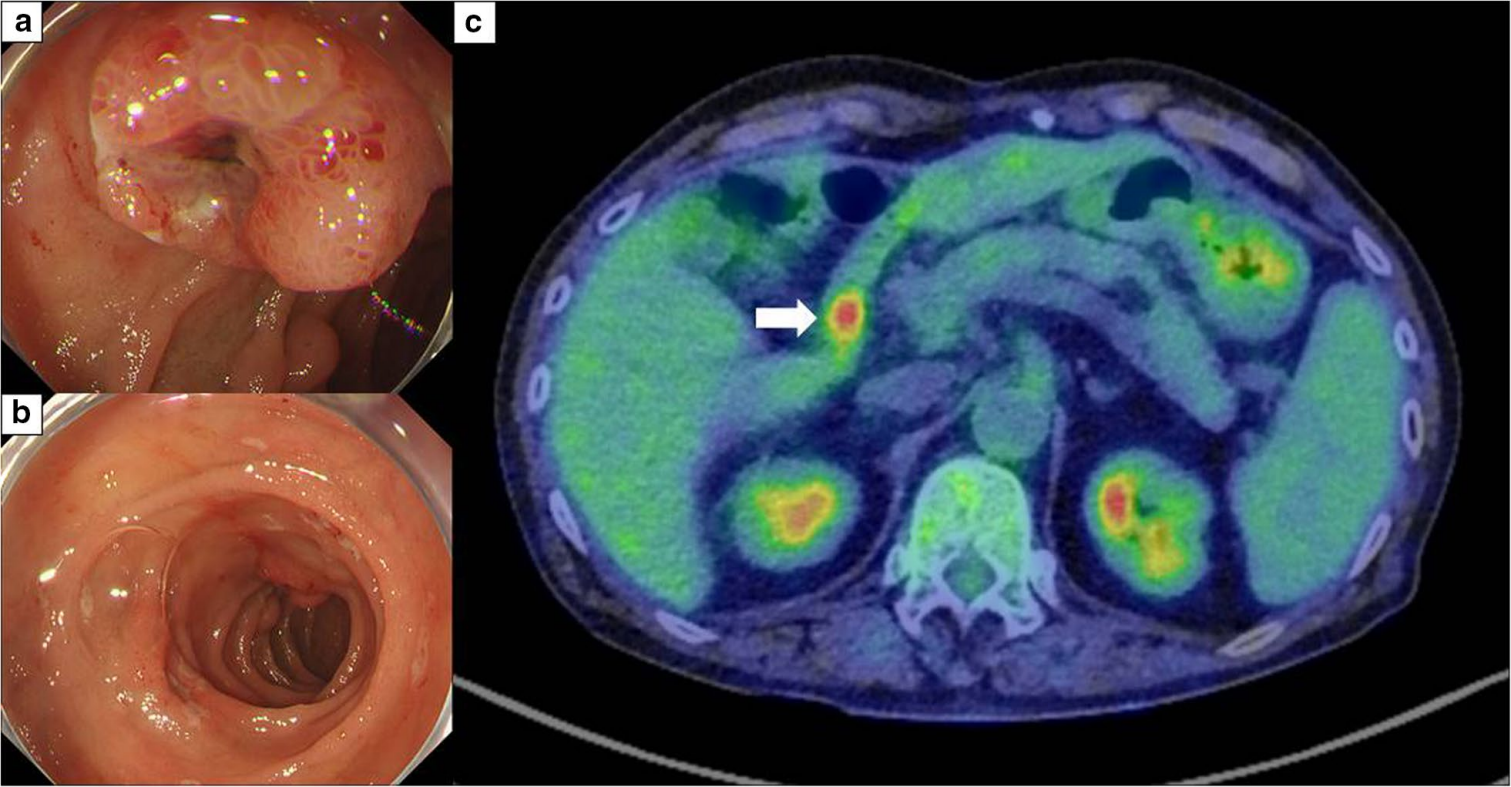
Preoperative examination. a, b Esophagogastroduodenoscopy (EDGS) images: EDGS detected two reddish elevated lesions with central ulceration in the duodenum. a The anal side tumor was reddish elevated lesions with central ulceration. The oral side tumor was ulcerative lesion with slightly elevated red border. c Positron emission tomography-CT (PET-CT) image: PET-CT showed increased uptake (mean maximum standardized uptake value [SUV_max_]: 6.25) of the oral side tumor in the duodenum
